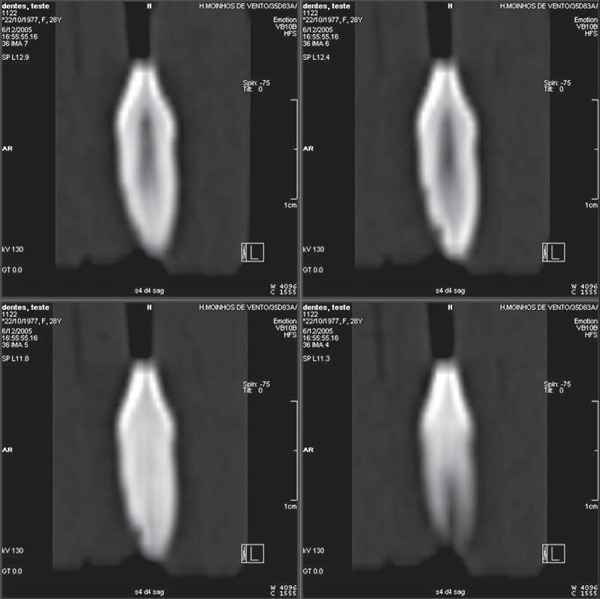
Series of sagittal CT sections showing cavities on apical and middle thirds
